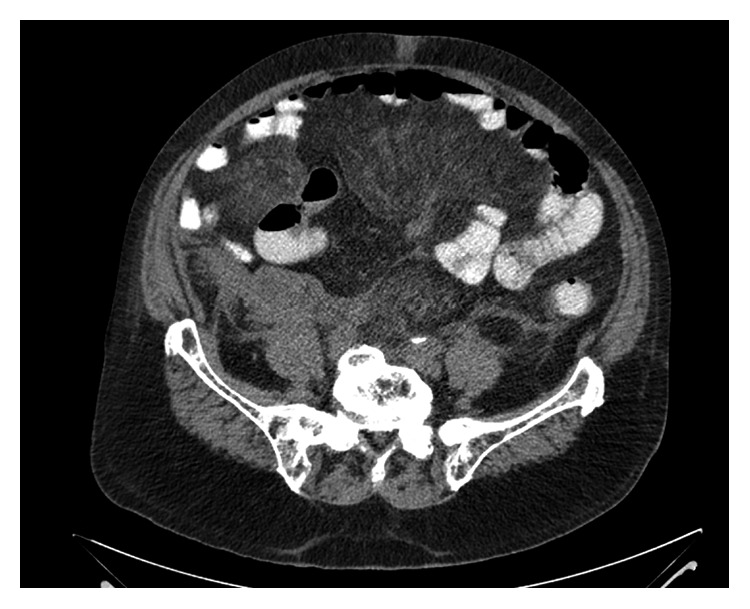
CT scan of the abdomen without contrast revealing mesenteric thickening with edema.
